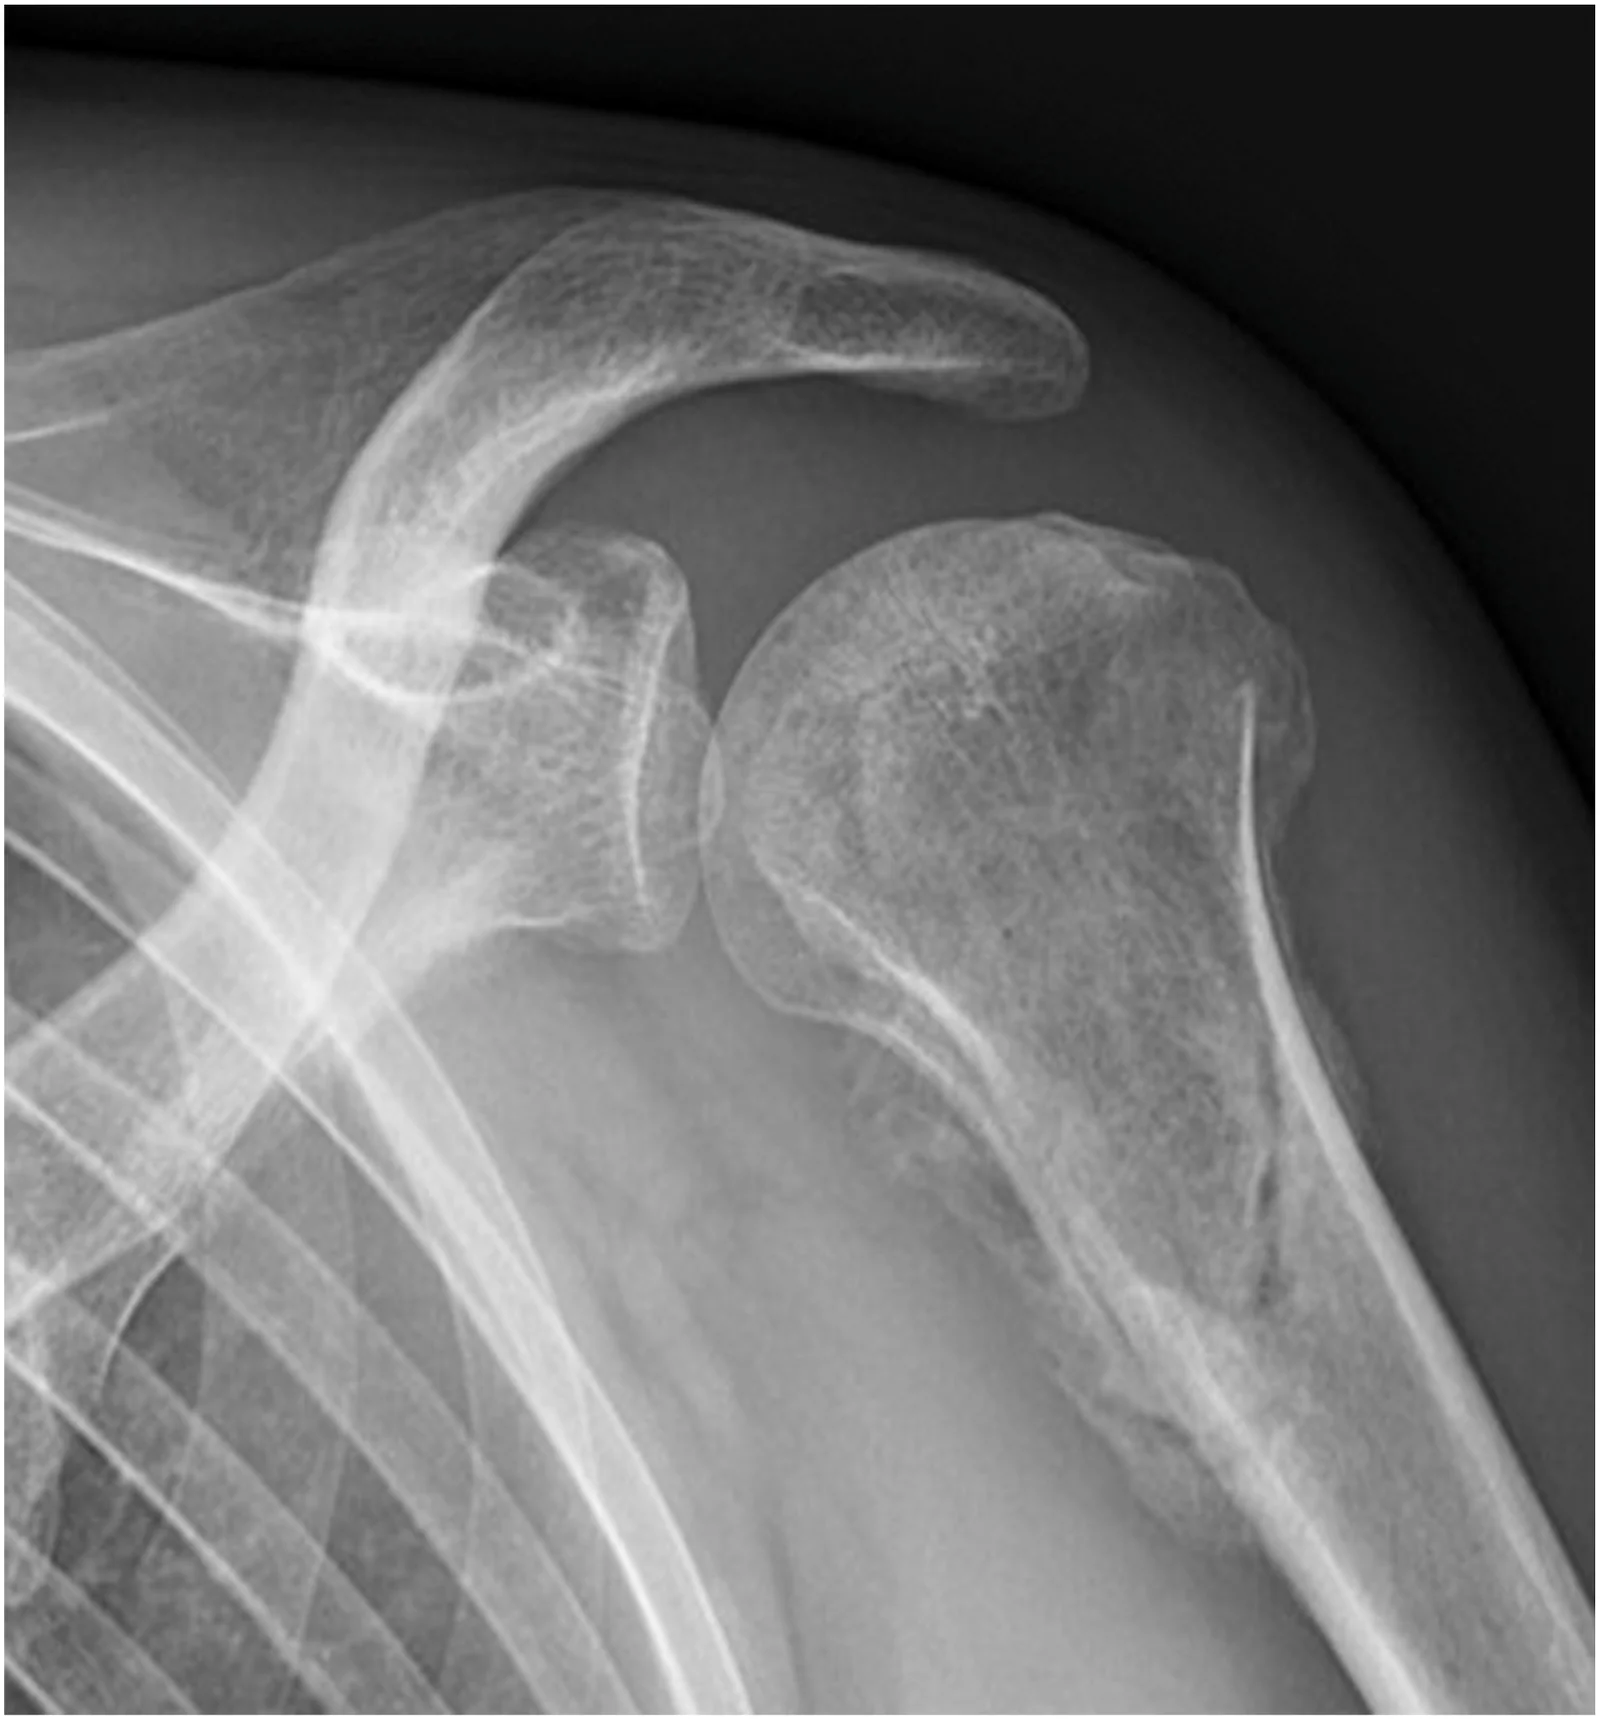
Conventional anteroposterior radiograph at 6 weeks demonstrated fracture union.
